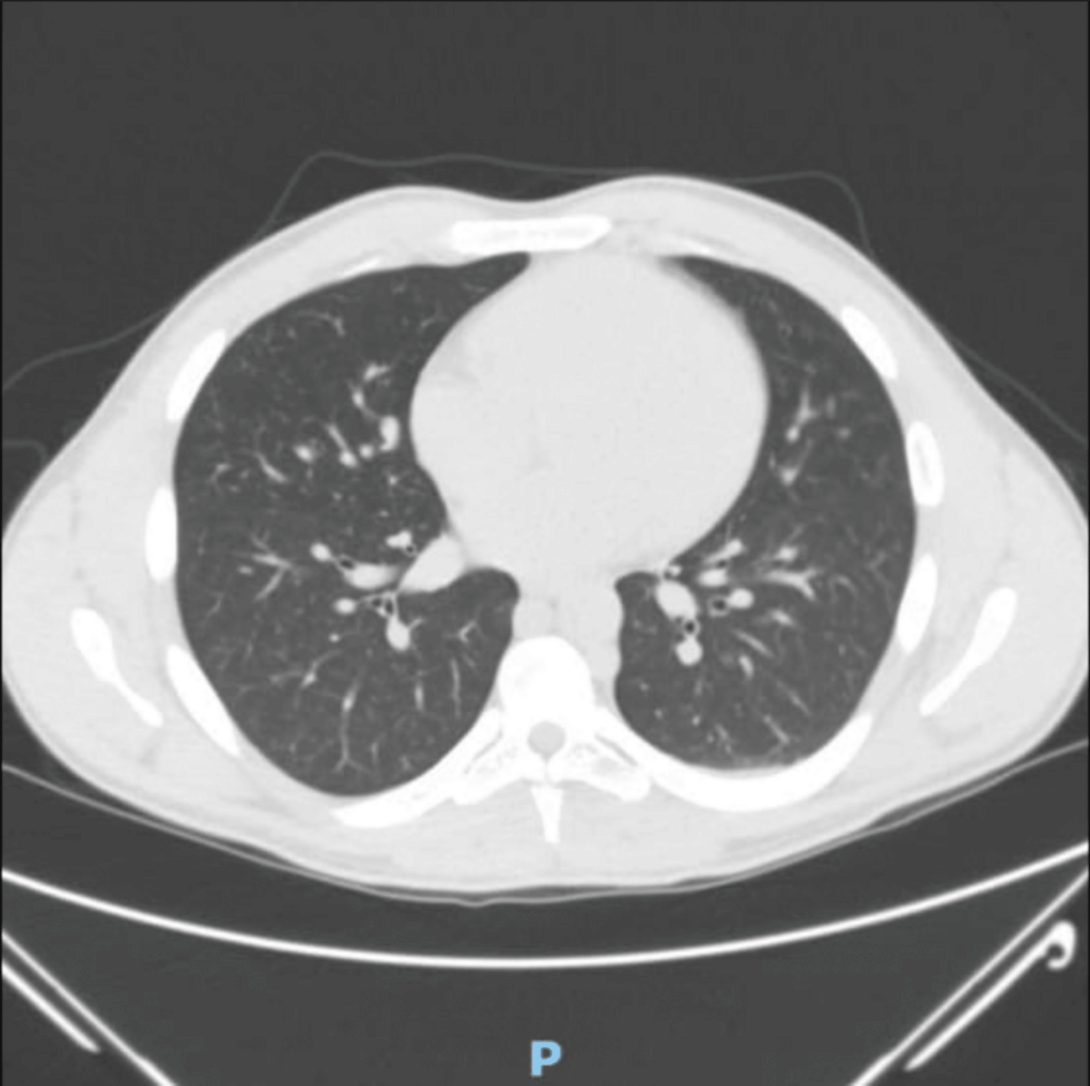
CT chest showing mild haziness
